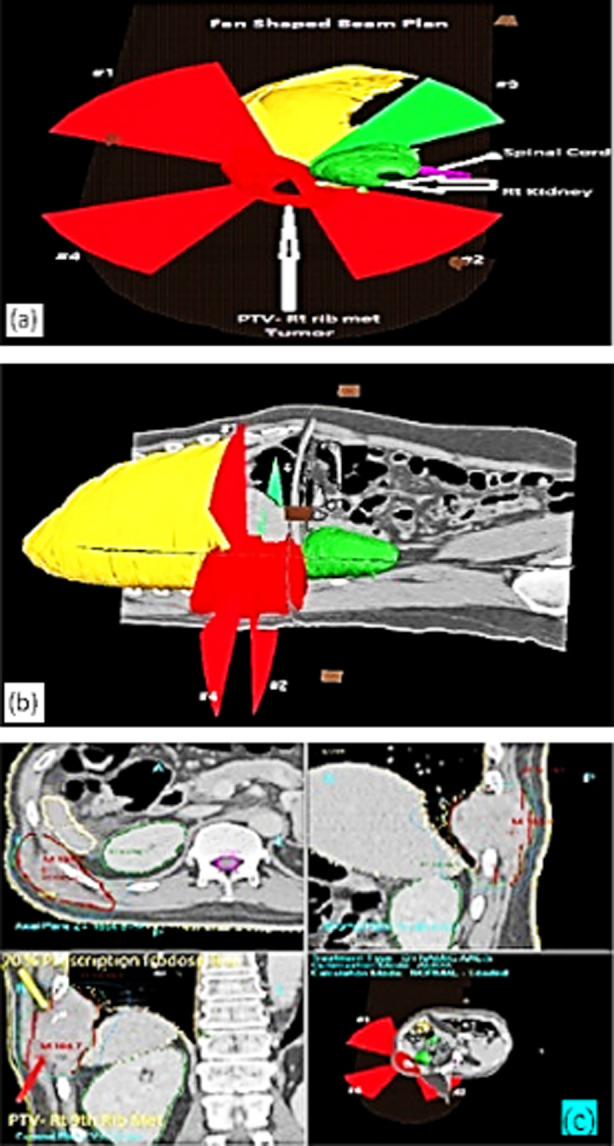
VMAT-SBRT, Fan shaped beam planning display: (a) Right rib metastases was planned for 10 Gy x 3 Fr @ 70% isodose line. (b) Planning with CT images (c) Three dimensional view.
